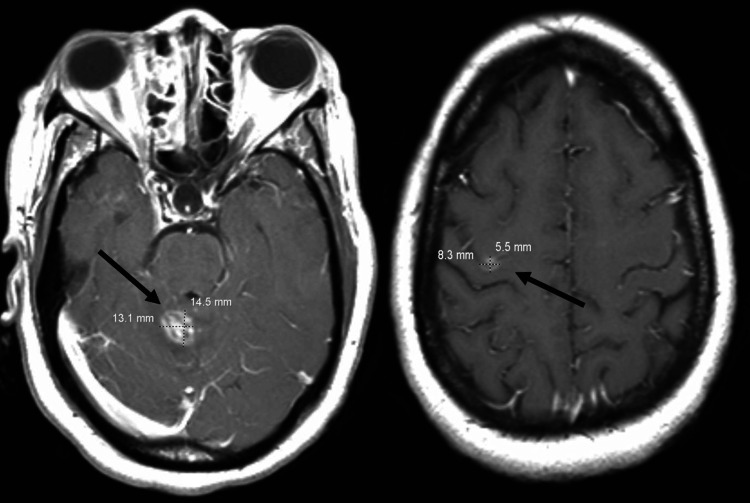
Multiplanar multisequential brain MRI image with and without contrast Imaging indicated a 1.5 x 1.3 x 1.4 cm right superior cerebellum enhancing lesion (left panel) with mild vasogenic edema as well as a 0.6 x 0.8 x 0.6 cm right frontal lobe lesion (right panel) MRI: magnetic resonance imaging
